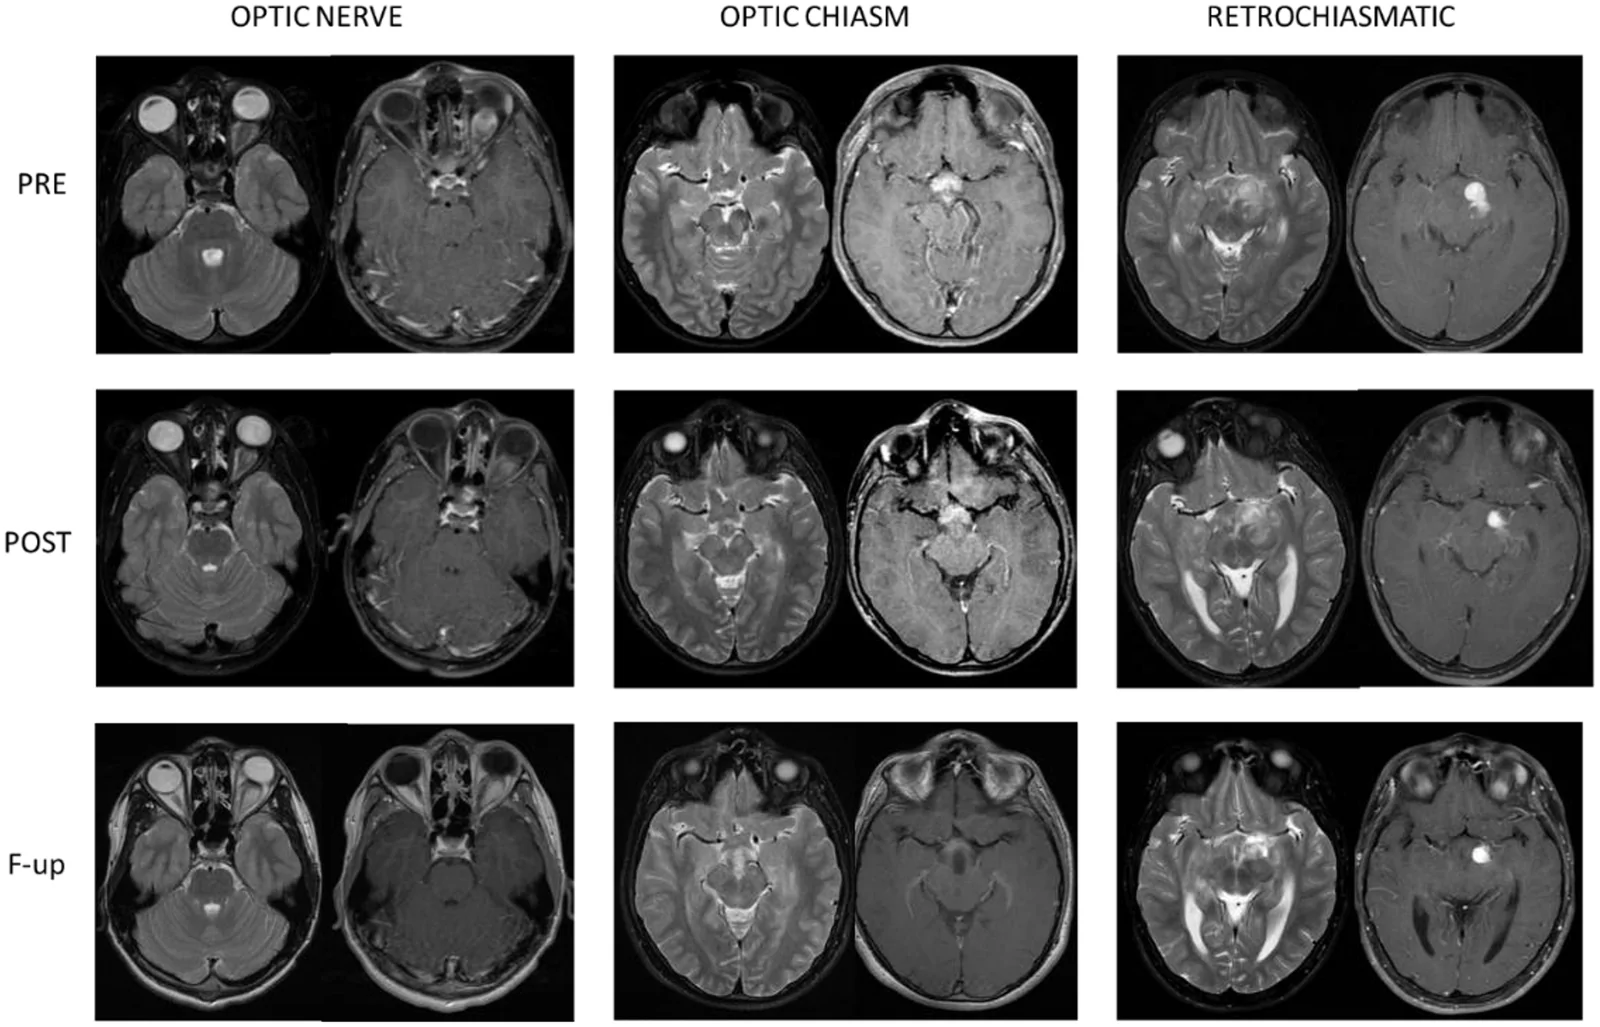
Brain MRI of OPG patients acquired at baseline (upper row), shortly after mNGF administration (middle row), and after four years of follow-up (lower row). For each site of involvement, 2D axial T2w Fast Spin Echo (FSE) sequence (left image) and 2D axial T1w FSE after contrast administration sequence (right image) are shown
